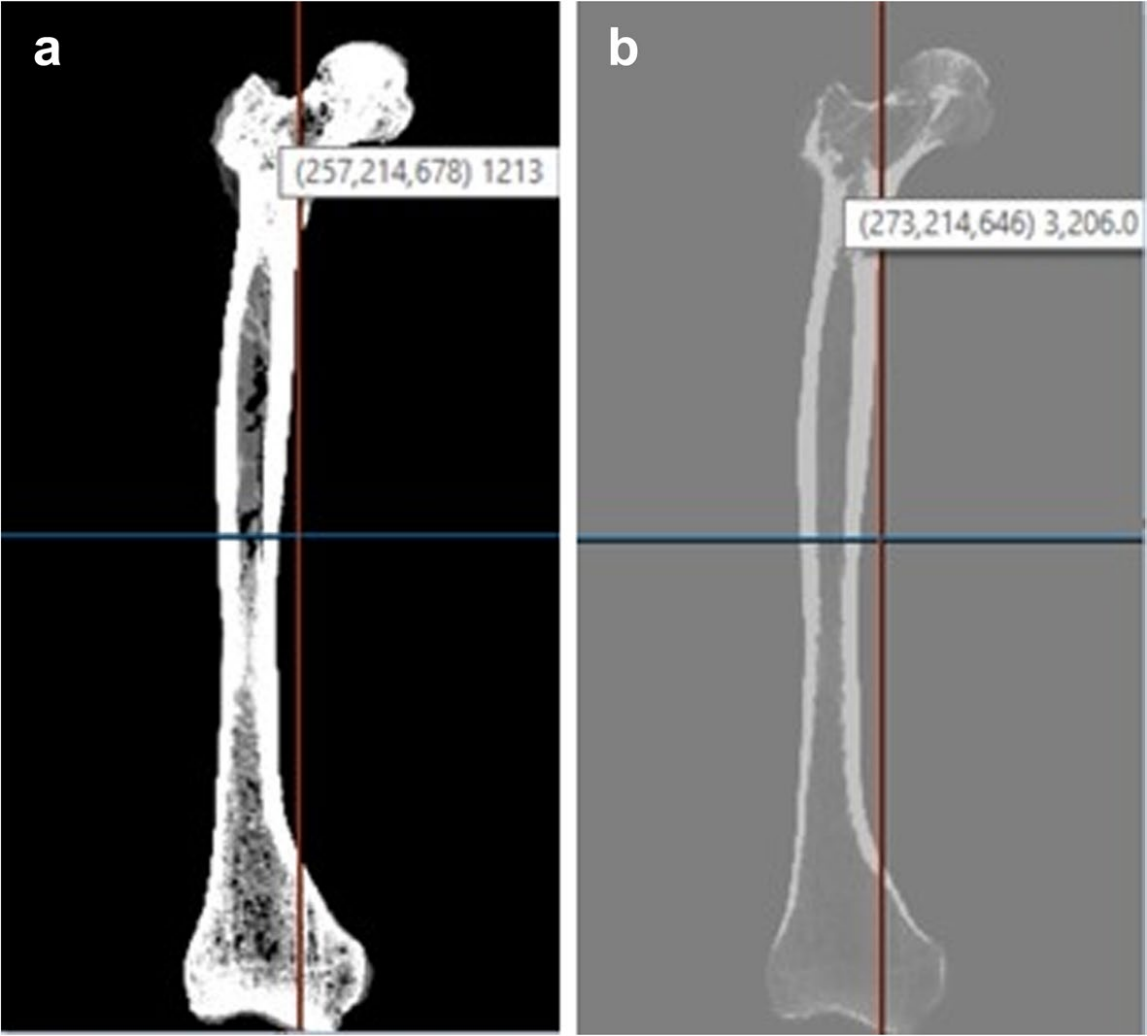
(a) Left. Original CT scan prior to processing using our PIP in the original grayscale (x,y,z, grayscale) (b) Right. CT scan after processing using our PIP in Young’s Modulus (MPa) (x,y,z, Young’s Modulus)
